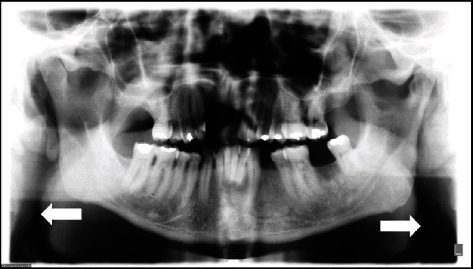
Panoramic radiograph showing the presence of bilateral radiopaque masses (arrows).
